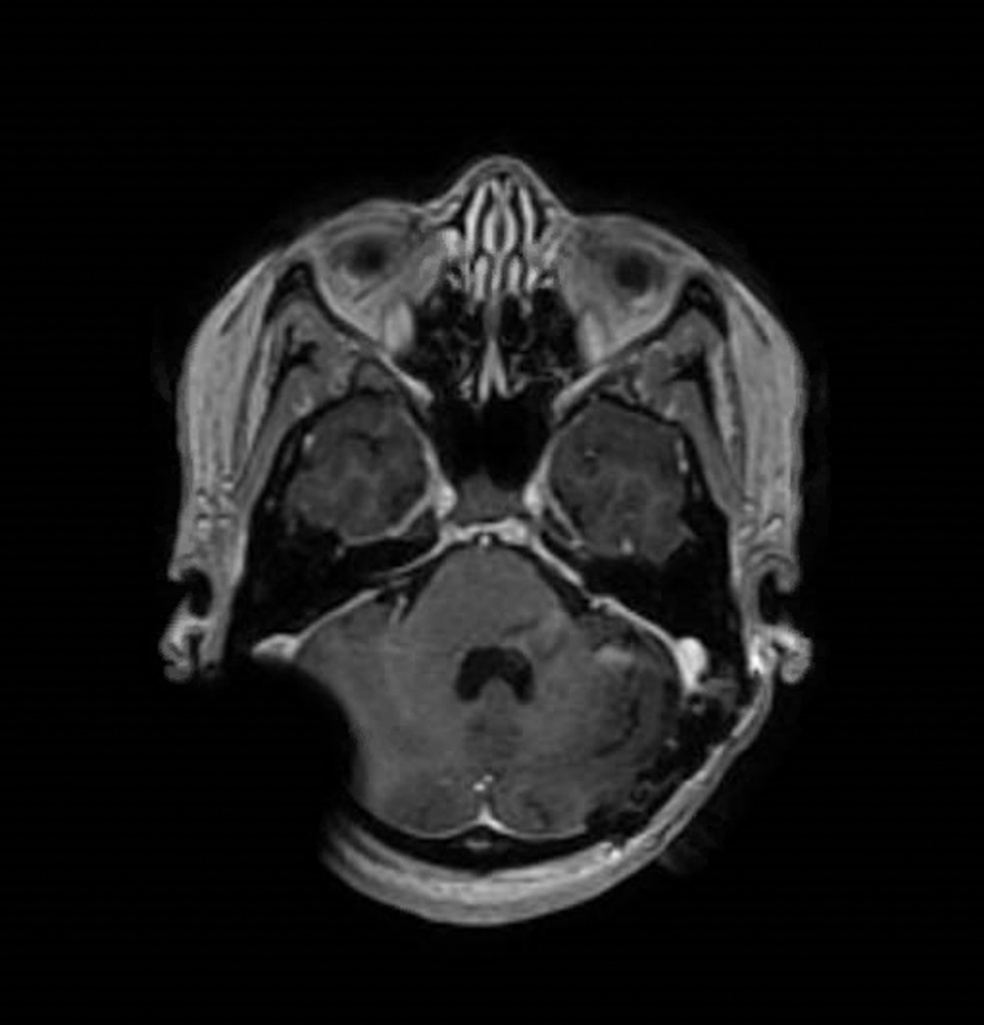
Contrast-enhanced MRI after completion of proton-beam radiotherapy shows complete resolution of the tumor.
